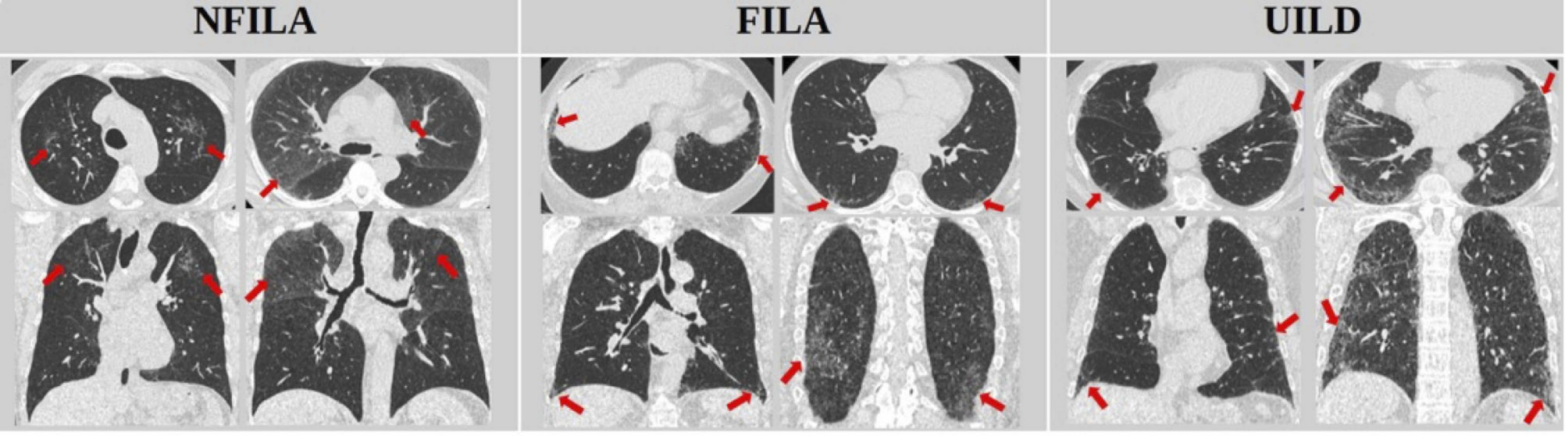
Axial and coronal CT examples of non-fibrotic interstitial lung abnormality (NFILA), fibrotic interstitial lung abnormality (FILA) and undiagnosed interstitial lung disease (UILD) identified in the SUMMIT cohort. NFILA required the presence of non-dependent ground glass opacities and/or reticulation with no associated traction bronchiolectasis evident in any lobes. FILA required traction bronchiolectasis (coexisting with reticulation with/ without non-dependent ground glass opacities) to be evident in a maximum of 2 lobes. UILD required traction bronchiolectasis (coexisting with reticulation with/ without non-dependent ground glass opacities) to be evident in more than two lobes.
